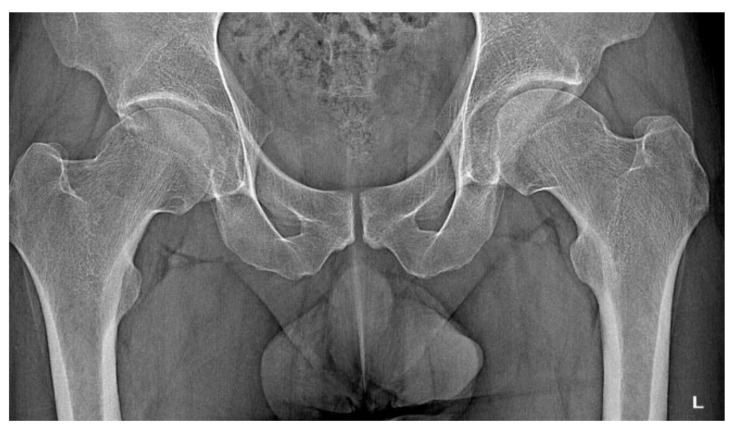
X-ray examination of the hip joints with the features of FAI.
